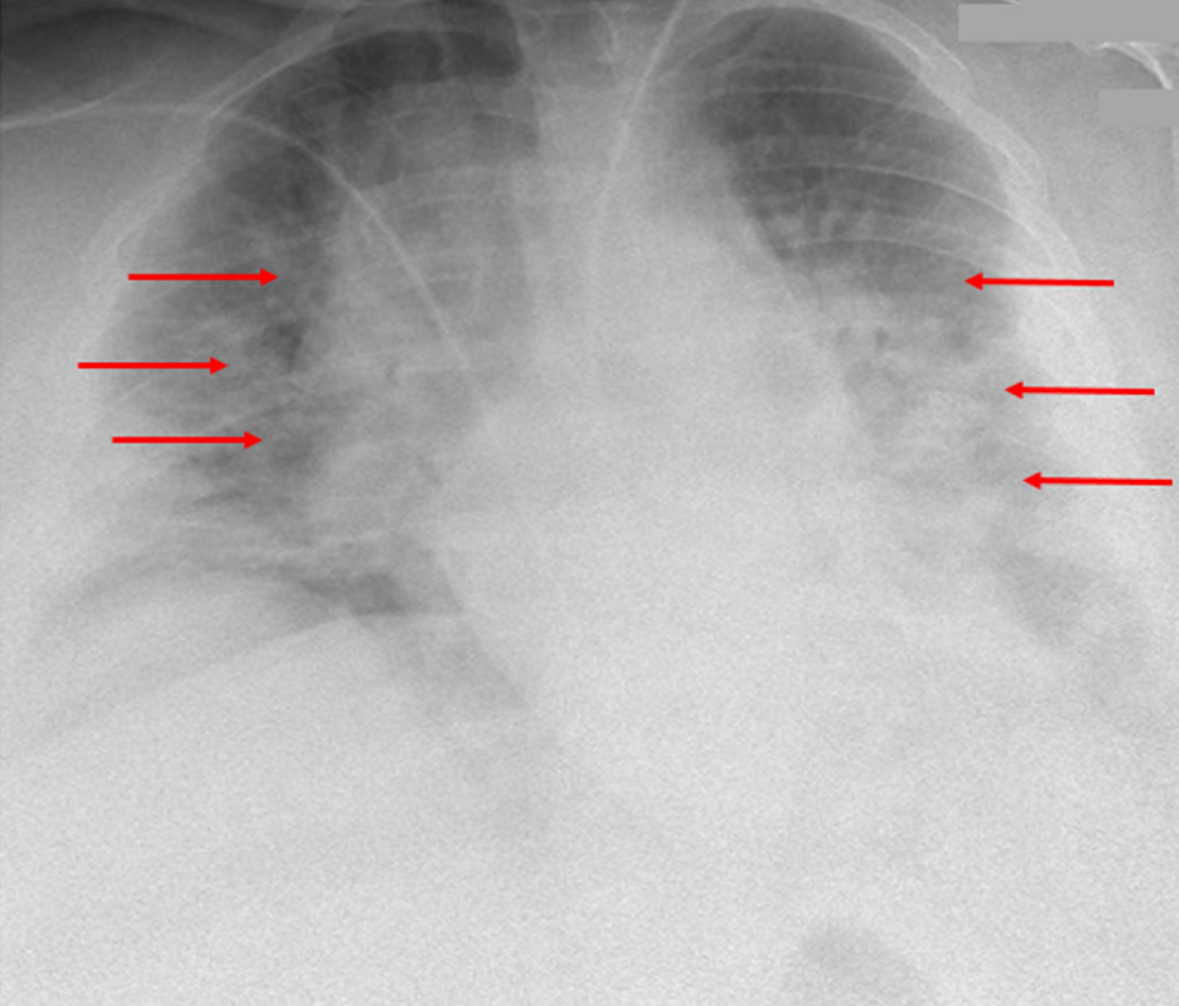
Initial chest radiograph An initial chest radiograph of the patient demonstrates diffuse and bilateral pulmonary opacities (red arrows), significant for acute respiratory distress syndrome. At this time, the etiology for acute influenza A virus is unknown, but the nidus of disease is suspected to be an infection.
